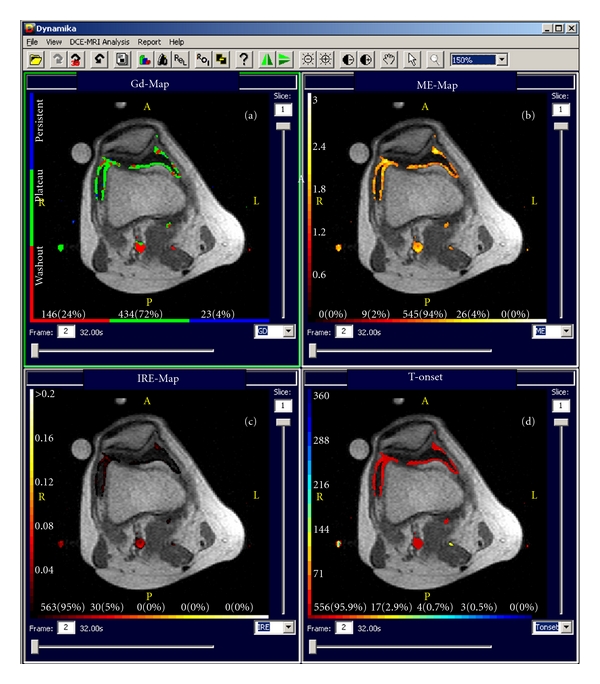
Parametric maps derived from the same DCE-MRI dataset reflecting the Gadolinium behaviour and distribution over time (Gd), maximum enhancement (ME), initial rate of enhancement (IRE), and time onset of enhancement (T-onset). The maps are derived from the baseline images of the case study representing a flair of moderate arthritis activity of the knee. Note the pulsation artefacts of the popliteal artery that give false “hot” points in the image related to a horizontal line of the artery.
